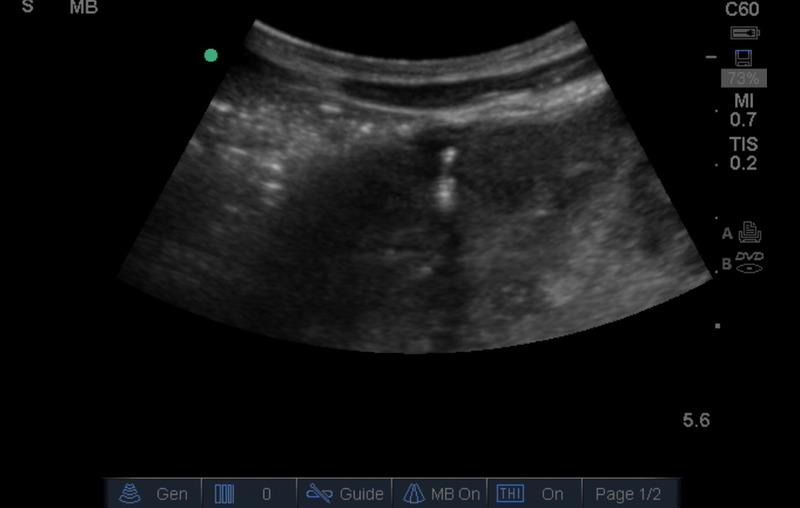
Ultrasound-guided pigtail placement in the left flank.
